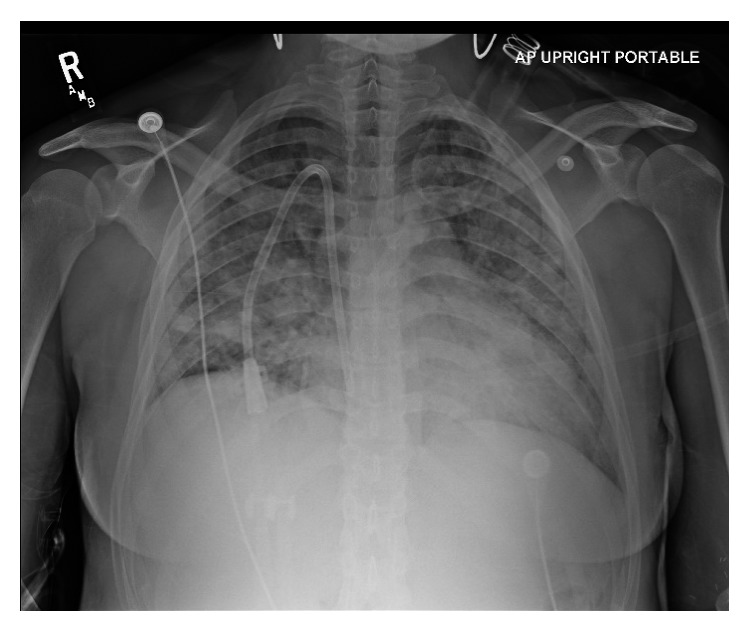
Portable chest radiograph demonstrates diffuse bilateral patchy opacities.
